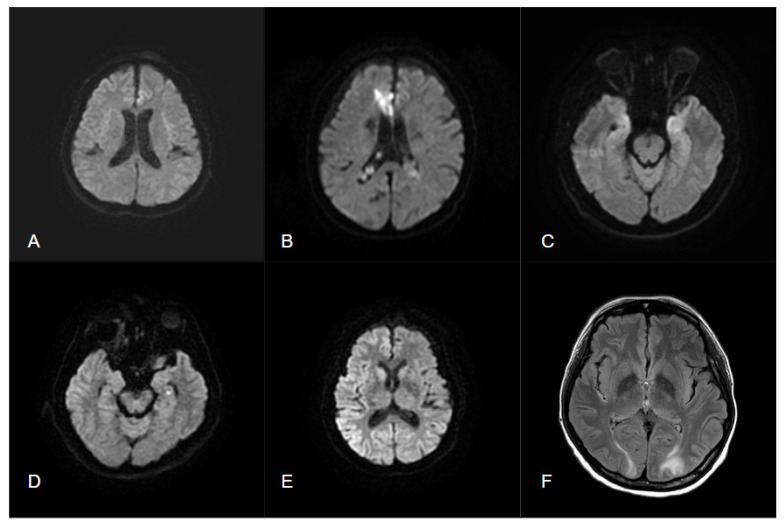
(A) Status epilepticus presents a left frontal cortical hyperintensity in DWI; (B) acute ischemic infarction in right ACA territory with DWI hyperintensity; (C) autoimmune encephalitis presents with bilateral hyperintensity in DWI and T2 FLAIR; (D) dot-like hyperintensity of DWI in TGA; (E) diffuse gyriform hyperintensity of DWI in cerebral cortex in sCJD; (F) bilateral hyperintensity of T2 FLAIR in white matter regions without abnormalities in DWI in PRES.
